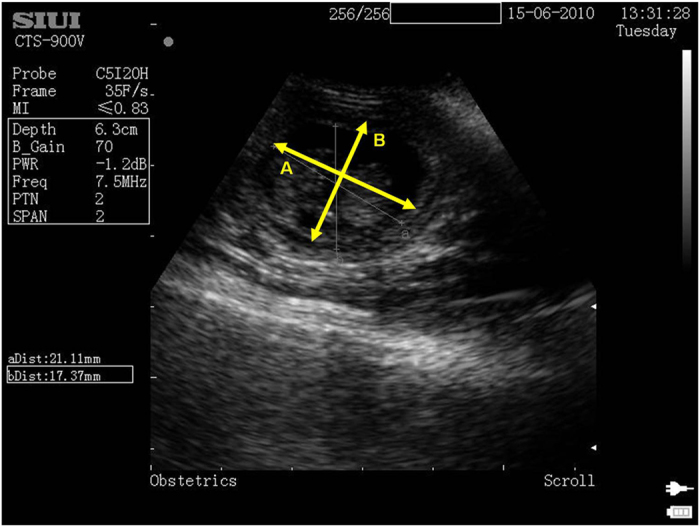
Cross-sectional ultrasound scan of individual fetus with 2 perpendicular measurements A and B used to calculate the cross-sectional area (A/2 × B/2 × π).
